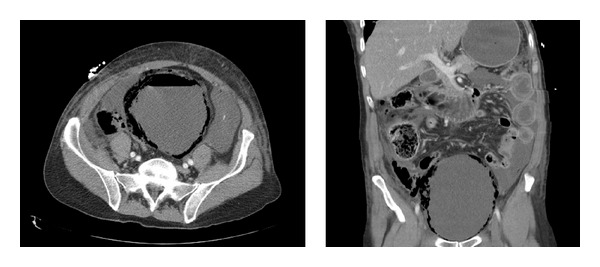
Computed tomography scan of the abdomen showed air in the bladder and the thickened bladder wall, associated with air intra- and retroperitoneally.
